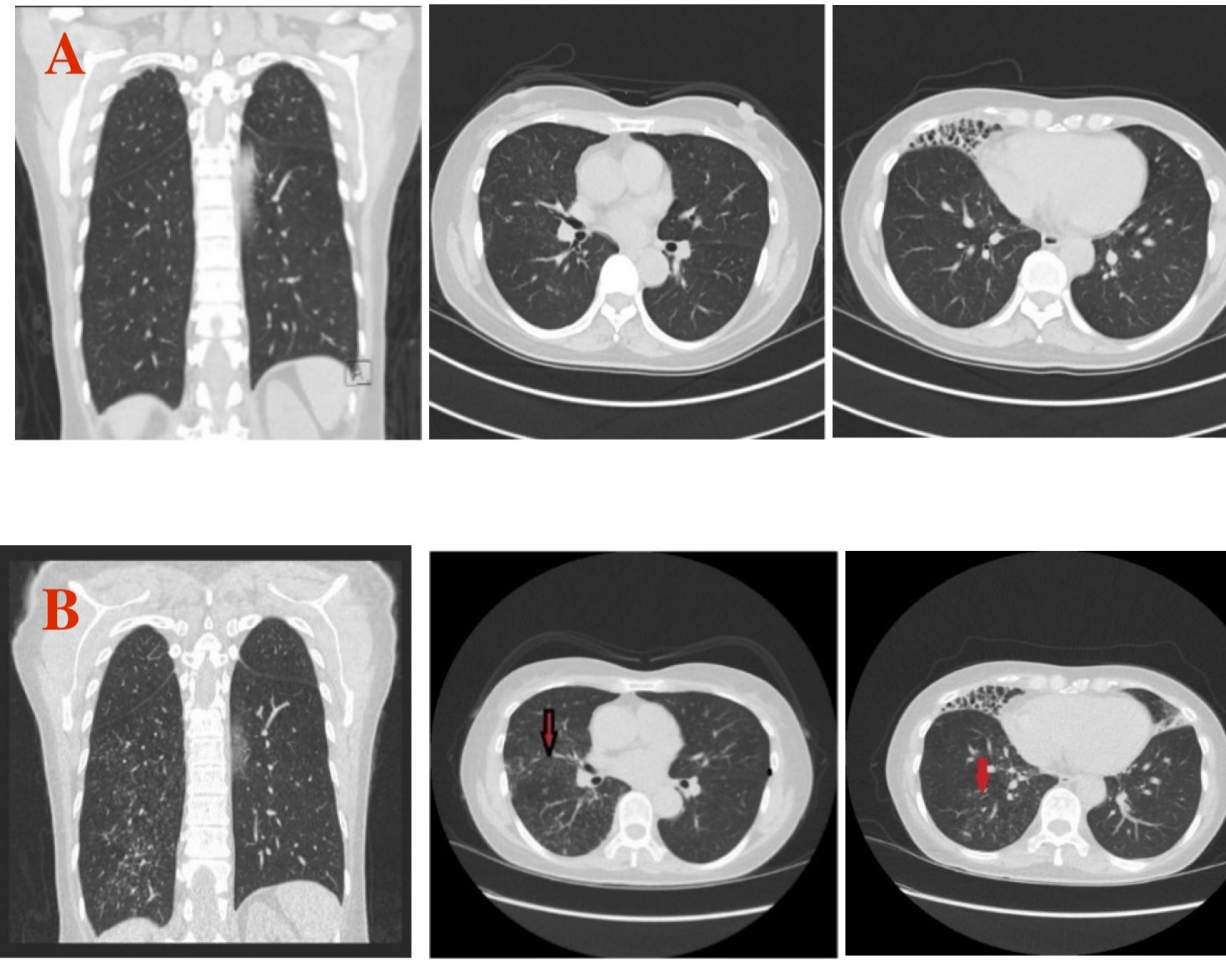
Features of *M. shinjukuense*. (A) Chest CT scan from April 2022. Left: coronal view. Middle: transverse view with mild nodularity in the right upper lobe. Right: bronchiectasis in the right middle lobe and mild nodularity in the right lower lobe. (B) Chest CT scan from August 2024. Left: worsening nodularity seen in the right middle and lower lobes. Middle: worse nodularity in the right upper lobe. Right: worse nodularity in the right lower lobe.
